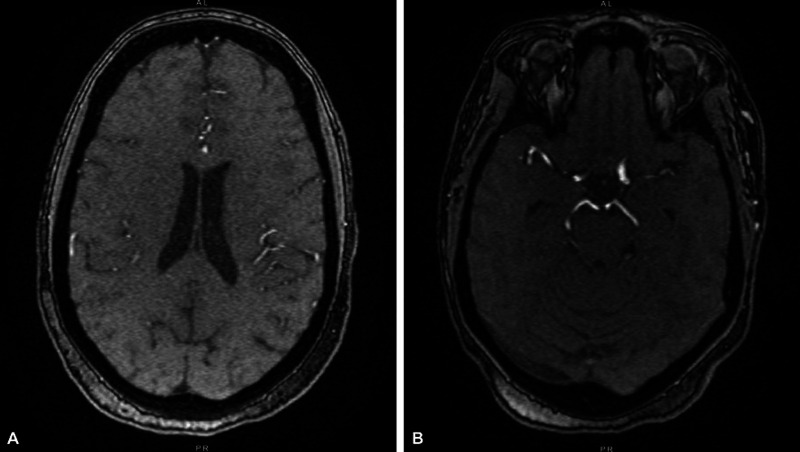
A. T1-weighted MRI of the brain without contrast at the patient’s second ED visit; B. MRA of the brain without contrast at the patient’s second ED visit A. This unenhanced MRI image of the brain is within normal limits. B. The circle of Willis had no evidence of major vessel stenosis, cutoff, narrowing, or aneurysmal dilatation. The vertebral arteries appear co-dominant. ED: emergency department; MRI: magnetic resonance imaging; MRA: magnetic resonance angiography
